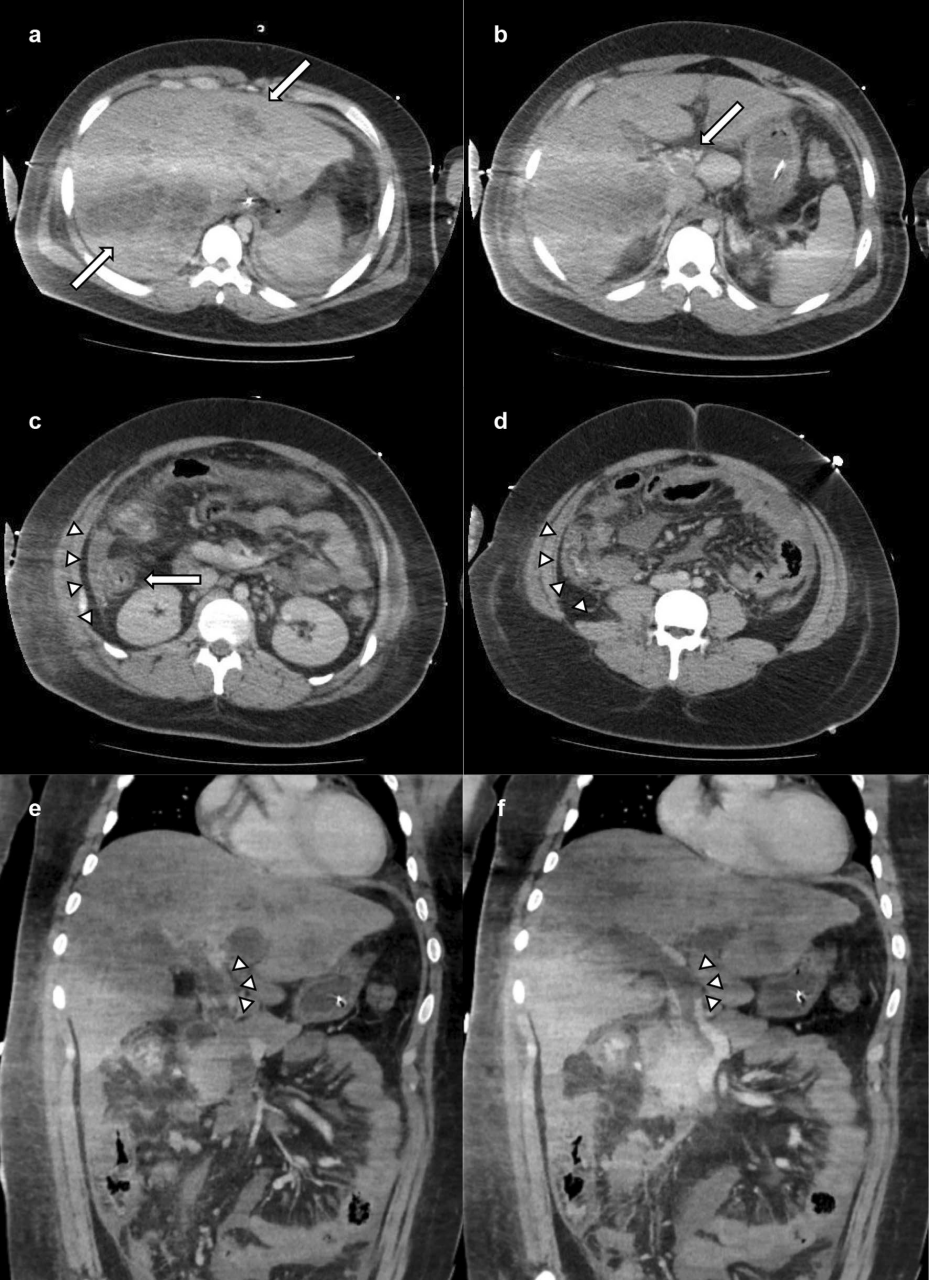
Contrast-enhanced CT at admission revealed a multiple bilobular ring-enhanced lesions in the liver (arrows), b a low-density area in the portal vein (arrow), c an enlarged appendix (arrow), and inflectional ascites in the right paracolic gutter (arrowheads), e and f a low-density area in the portal vein of the sagittal sections (arrowheads). CT: computed tomography
